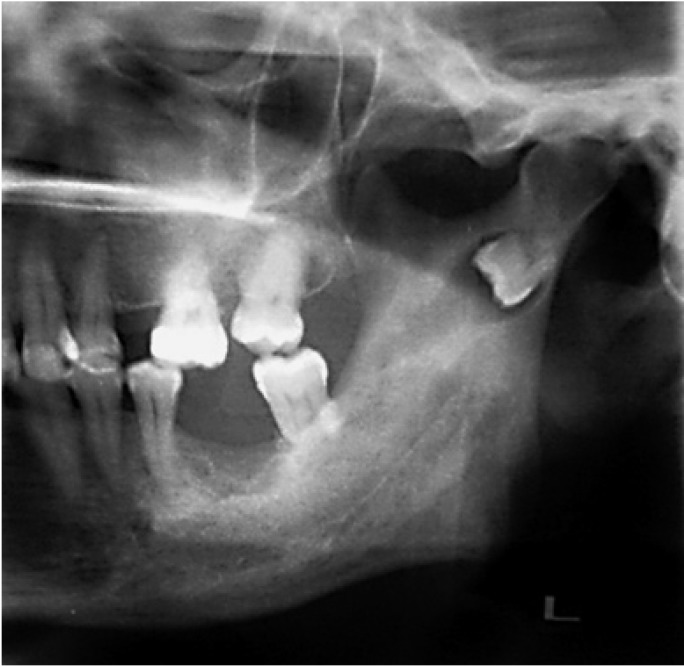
Panoramic radiograph showing an inverted third molar associated with a radiolucent image in the left subcondylar region.
